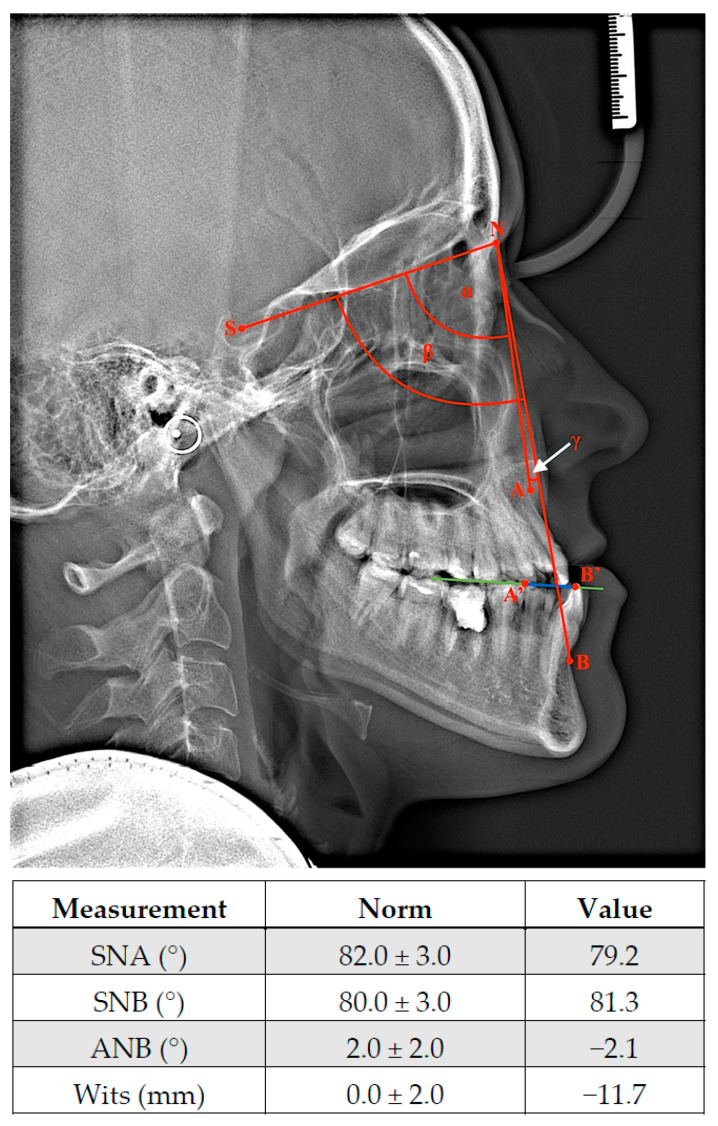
Representative cephalometric X-ray and part of cephalometric analysis according to Segner and Hasund presenting patients diagnosed with skeletal class III malocclusion before treatment. A-SNA; β-SNB; γ-ANB (white arrow); A’-B’0Wits (blue line); green line-occlusal plane. See the details description in text and Supplementary Materials section (Table S1) for all cephalometric measurements.
